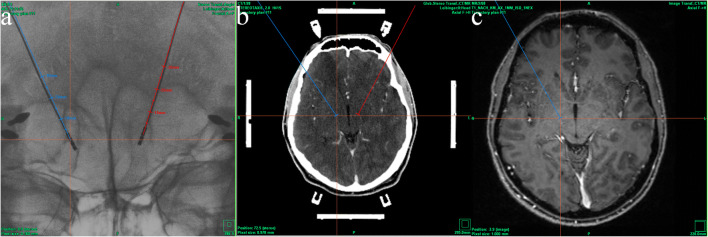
Co-registration of intraoperative stereotactic X-ray image (a) with stereotactic CT image (b) and preoperative MRI image (c)
